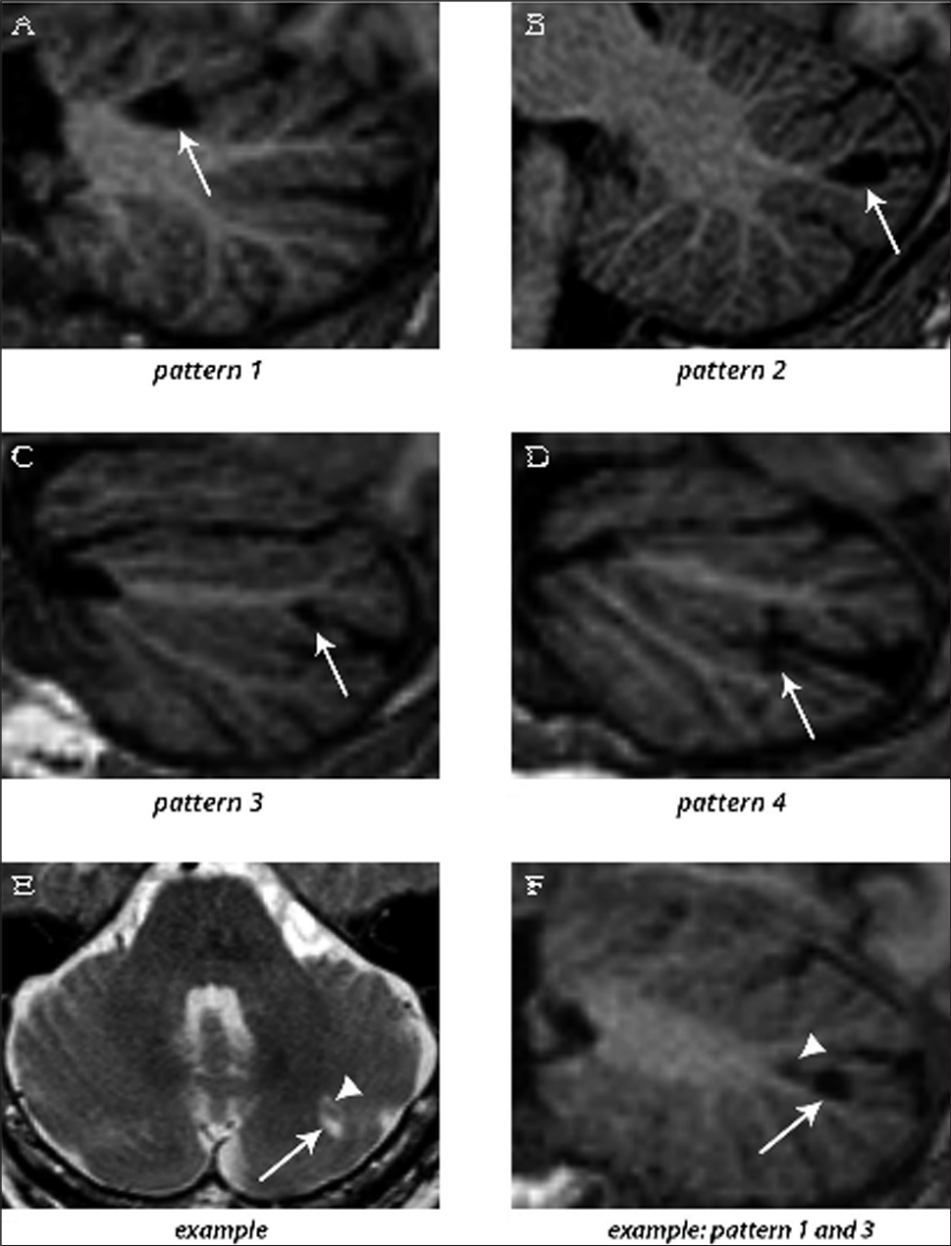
3D T1-weighted images (sagittal reconstructions) of the brain (A–D and F), providing excellent contrast between gray matter and white matter, show the four patterns by which cerebellar infarcts (arrows) typically affect the cerebellar cortex. An example of two cerebellar infarcts adjacent to each other (E; arrow and arrowhead) are detected on transverse T2WI. Sagittal reconstruction of 3D T1WI of the same two infarcts (F) shows how the topography of one infarct corresponds to pattern 3 (arrow), while the other infarct (arrowhead) corresponds to a small pattern 1 infarct. Notice the sparing of the subcortical and deep white matter in each infarct. The images are cropped to only display the cerebellum. Reproduced from *Neuroimage: Clinical* [5].
